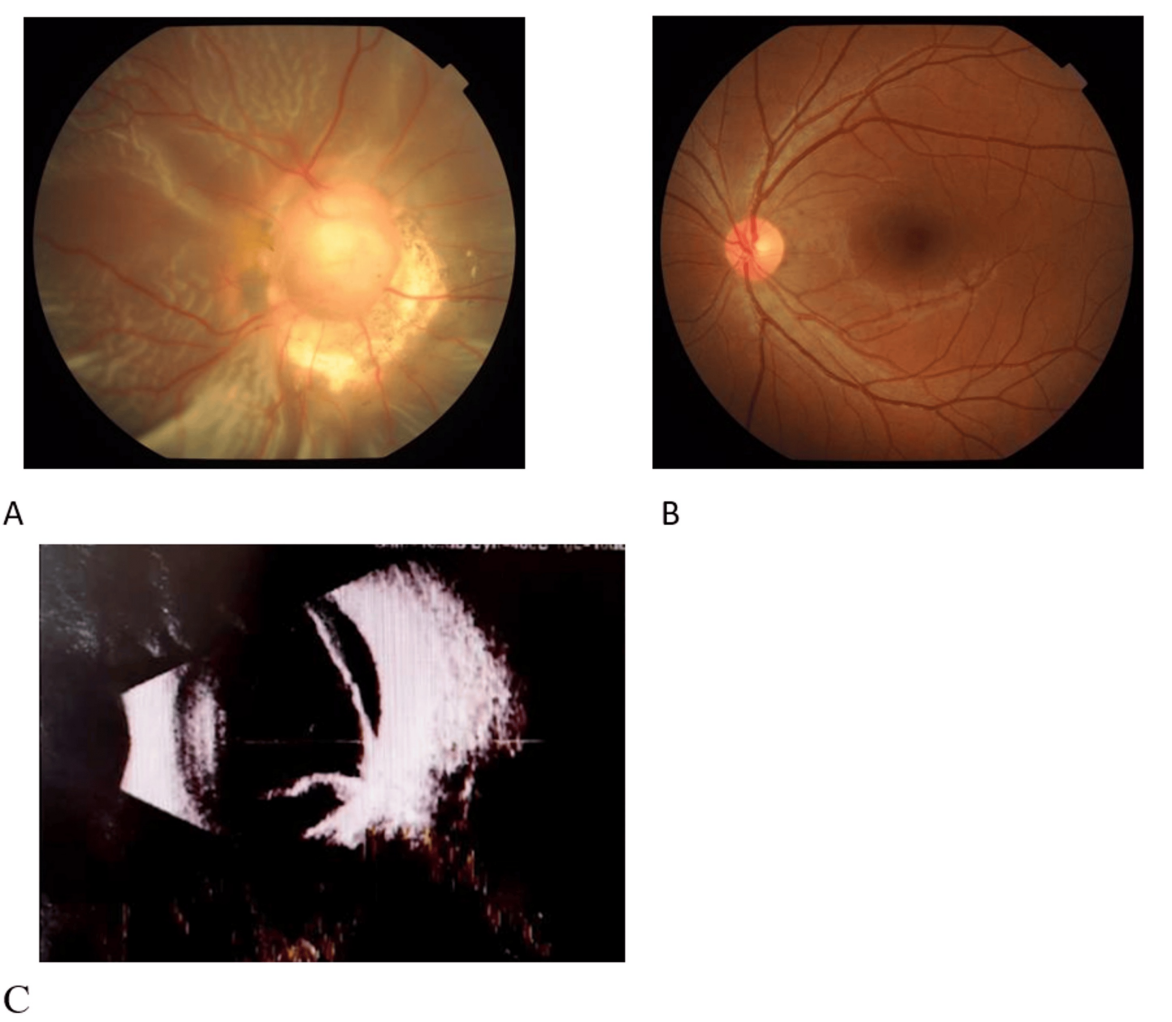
Preoperative ocular examination (A) Preoperative: Fundus Photography: The optic disc of the right eye appears large with a deep depression, covered by a grayish-white glial tissue on its surface, obscuring the vascular pattern within the optic disc. Several straight blood vessels emanate radially from the disc margin, making it difficult to distinguish arteries from veins. There is total retinal detachment. (B) The fundus of the left eye shows no significant abnormalities. (C) B-scan Ultrasonography of the Right Eye: Total retinal detachment is present, exhibiting a "funnel-shaped" alteration.
